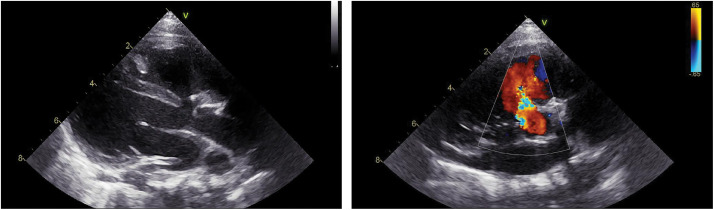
Preoperative transthoracic echocardiogram of patient 1. The echocardiogram shows a perimembranous VSD and dilated LV and RV. LV, left ventricle; RV, right ventricle; VSD, ventricular septal defect
